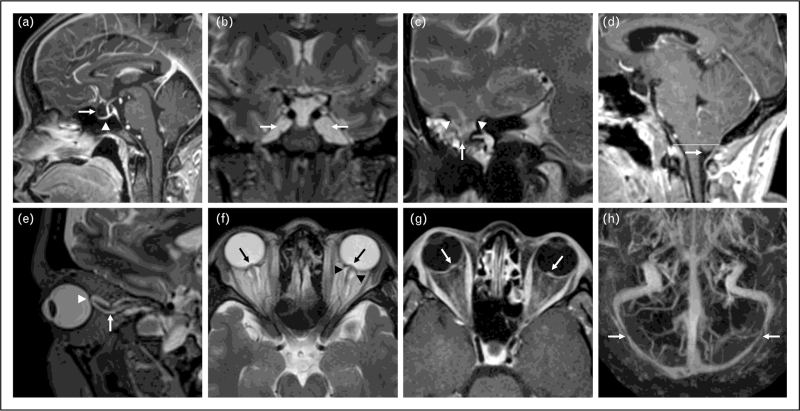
Imaging examples of radiological signs of intracranial hypertension. (a) Sagittal postcontrast T1-weighted image demonstrating an expanded, cerebrospinal fluid (CSF)-filled ‘empty’ sella turcica (arrow) and flattened appearance of the pituitary gland against the floor of the sella turcica (arrowhead). (b) Coronal T2-weighted image demonstrating abnormally enlarged Meckel caves bilaterally, filled with CSF (arrows). (c) Coronal T2-weighted image through the right temporal lobe demonstrating a meningoencephalocele. Defects in the tegmen mastoideum (margin indicated by arrowheads) transmit CSF and a portion of the right inferior temporal lobe (arrow). Fluid within adjacent mastoid air cells indicates associated CSF leak. (d) Sagittal postcontrast T1-weighted image demonstrating cerebellar tonsillar ectopia. The cerebellar tonsils, with normal rounded morphology (arrow), are in a low-lying position 5 mm below the foramen magnum (white line). (e) Oblique sagittal reconstruction of volumetric T2-weighted image in the plane of the optic nerve demonstrates posterior scleral flattening (arrowhead) and increased vertical tortuosity of the intra-orbital optic nerve sheath complex (arrow). (f) Axial T2-weighted image shows protrusion of the optic nerve head (arrows) and distention of the optic nerve sheaths by CSF (arrowheads) bilaterally. (g) Axial postcontrast T1-weighted image demonstrates enhancement of the optic nerve head bilaterally (arrows). (h) Cranio-caudal maximum intensity projection (MIP) of a postcontrast MR venogram. Arrows demonstrate smooth tapered severe stenosis of the distal transverse sinuses bilaterally.
